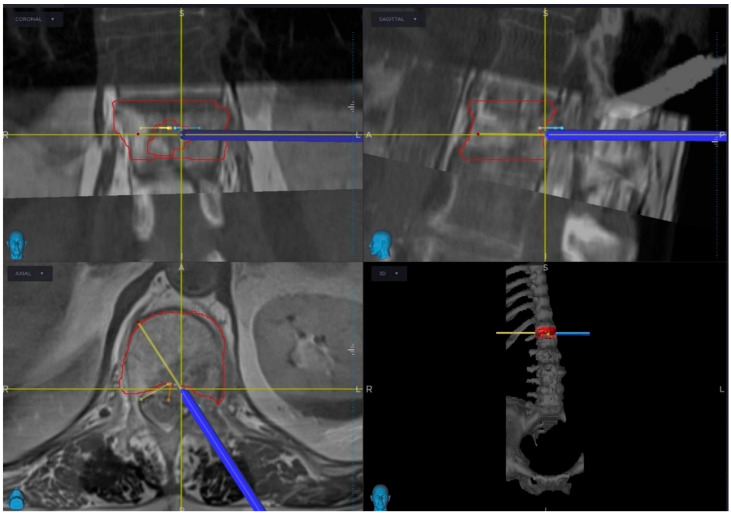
Intraoperative manual imaging merging of pre-operative standard lumbar MRI with the registered SBRT target plan and 3D-CT scan acquired with O-Arm Medtronic, after positioning of the D12 spinous passive frame. The pointer identifies the left trans-pedicular resection trajectory to perform Separation Surgery.
